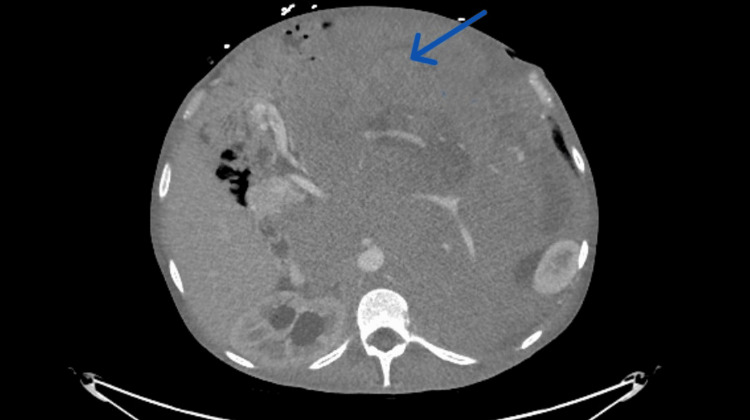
CT of the abdomen and pelvis with contrast showing very large retroperitoneal mass in the abdomen (blue arrow)
